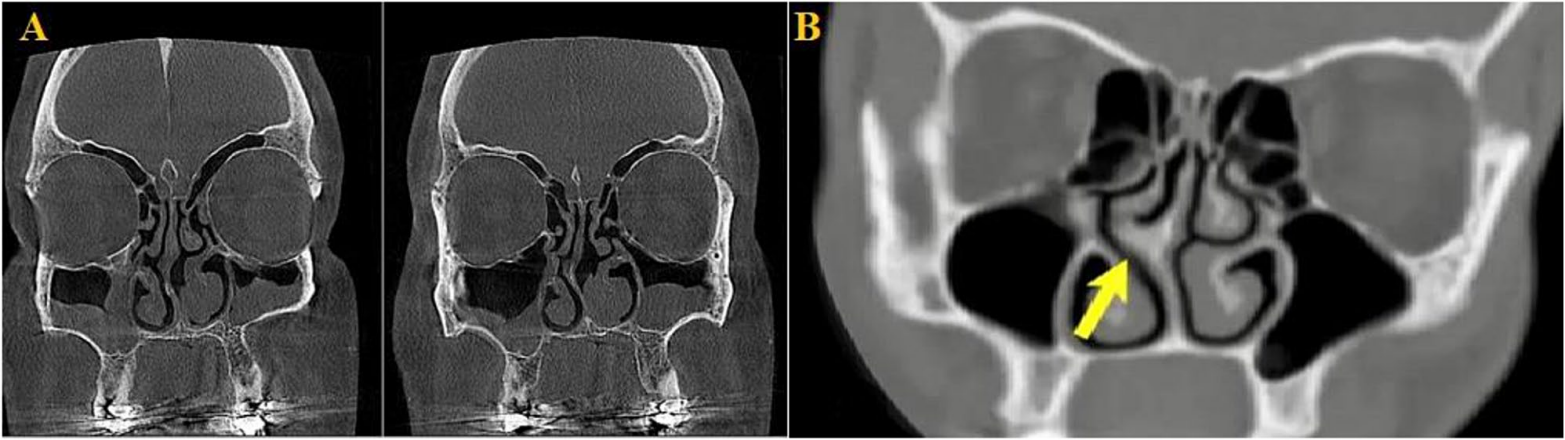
(A): NSD in CBCT; (B): NSD in MDCT
